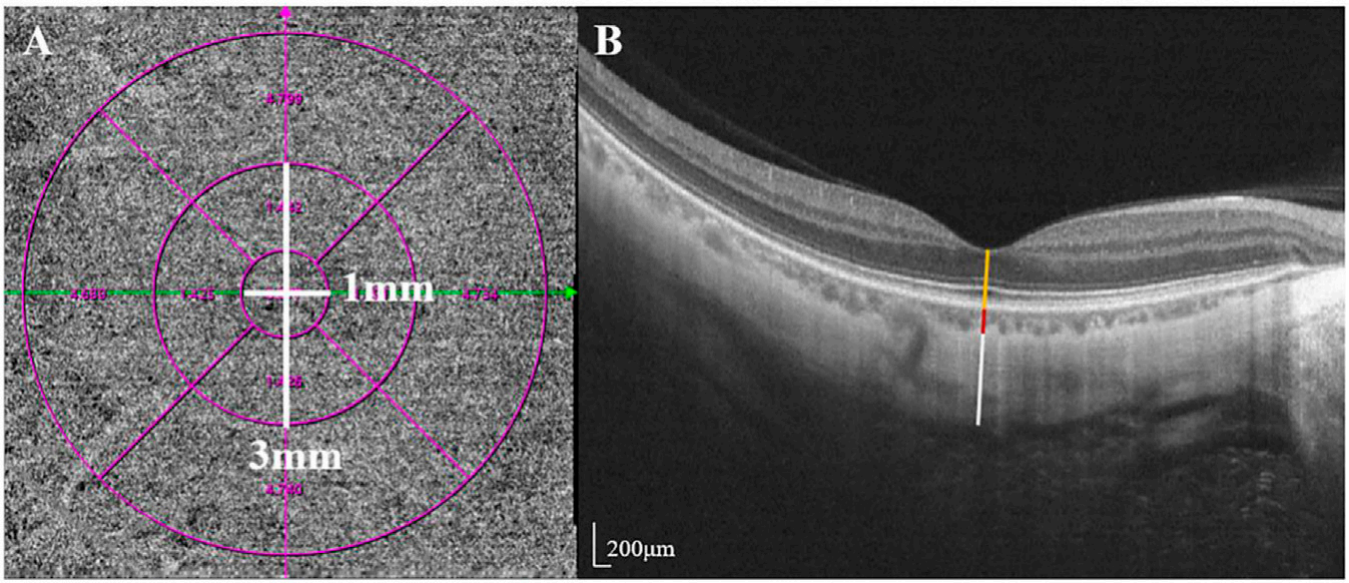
Two areas of macular and manually measured parameters (RT, CT, and ST). (A) The foveal region was a circular area with a diameter of 1 mm, centered on the fovea. The parafoveal region was a ring area around the fovea with an inner diameter of 1 mm and an outer diameter of 3 mm (B) Measurement of SFRT (yellow line), SFCT (red line) and SFST (white line). RT was defined as the vertical distance between the inner limiting membrane and the retinal pigment epithelium. CT was defined as the vertical distance between the outer edge of the retinal pigment epithelium (RPE) and the outer edge of the choroid. ST was defined as the vertical distance between the outer edge of the choroid and the outer border of the sclera.
